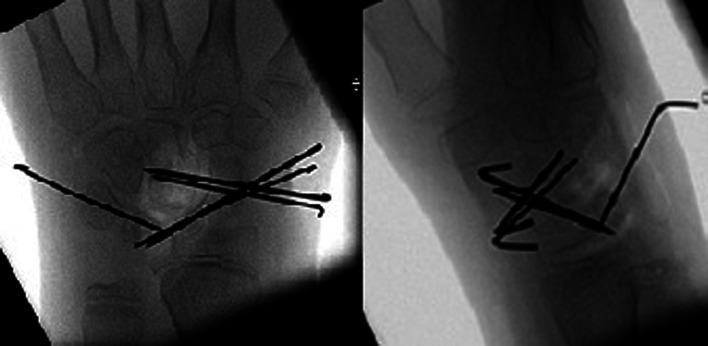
Postsurgery X-ray: carpal bones reduced and fixed with k-wires.
